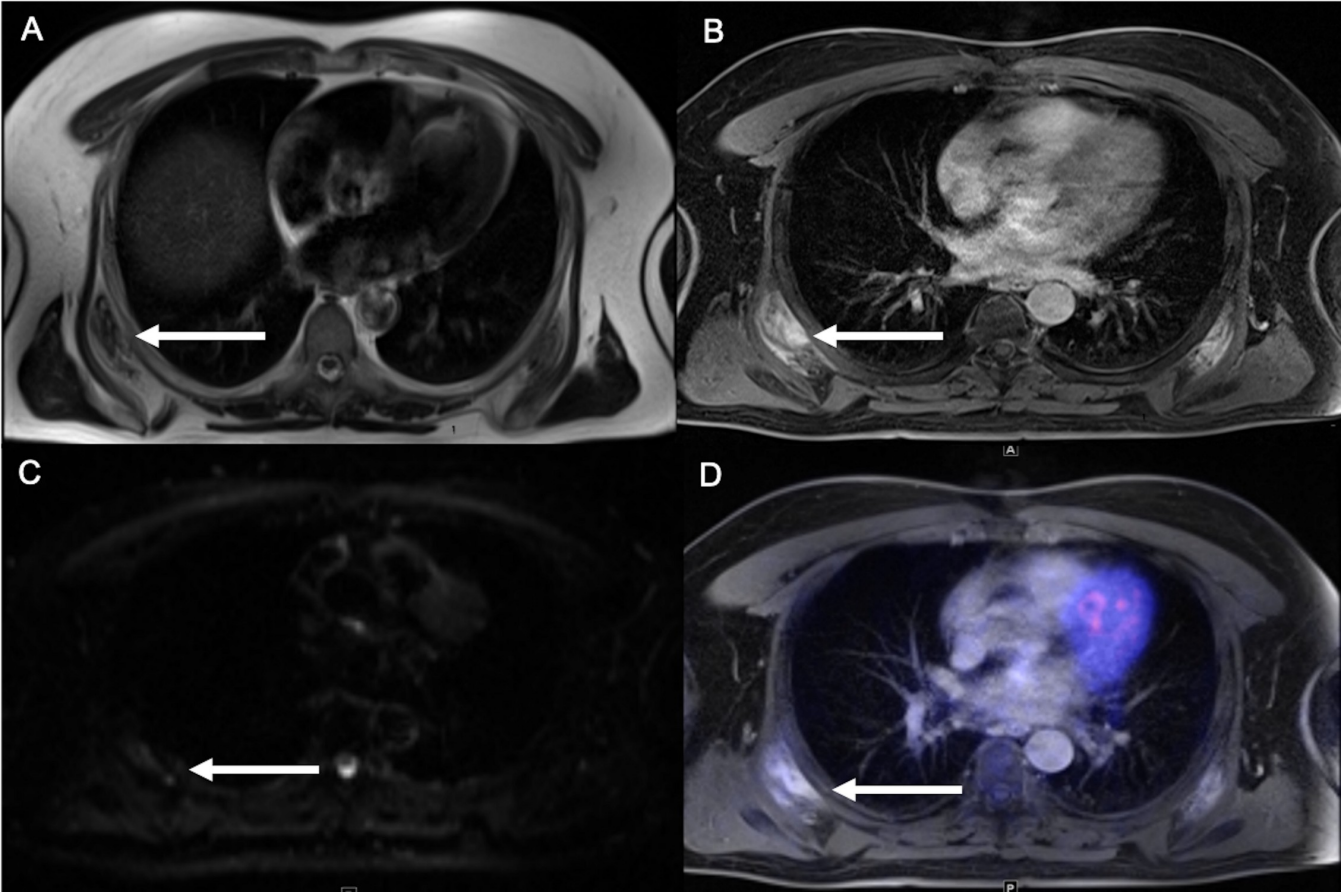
59-year old patient with suspicious subscapular lesions with isointense signal on T2w (A), intense inhomogeneous contrast enhancement (B), no diffusion restriction (C) and mild tracer uptake with SUVmax of 3.2 (D).
